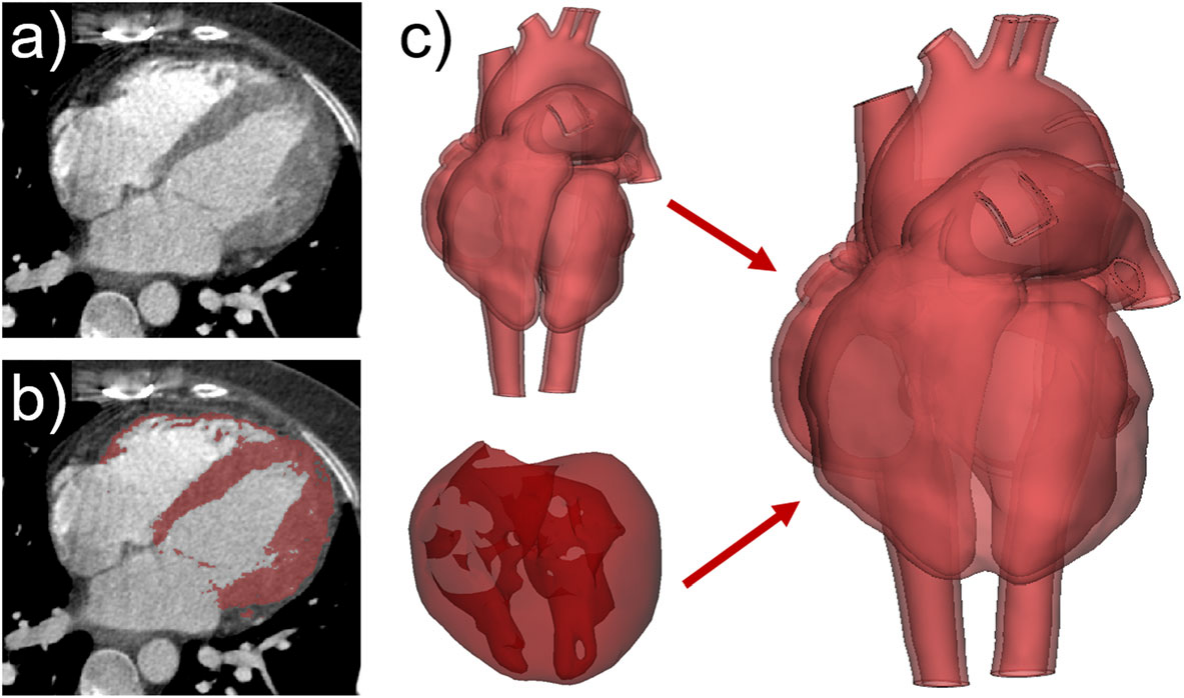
Boolean operations as essential part of the design process of 3D heart models including the myocardium. a Heart muscle visible on axial CT scan. b Segmentation of the heart muscle (red) performed by local thresholding techniques. c Combination of the hollow blood volume and the myocardium by a series of Boolean operations maintaining the correct anatomical intracardiac structure of the ventricles as well as the smooth outer surface of the myocardium
